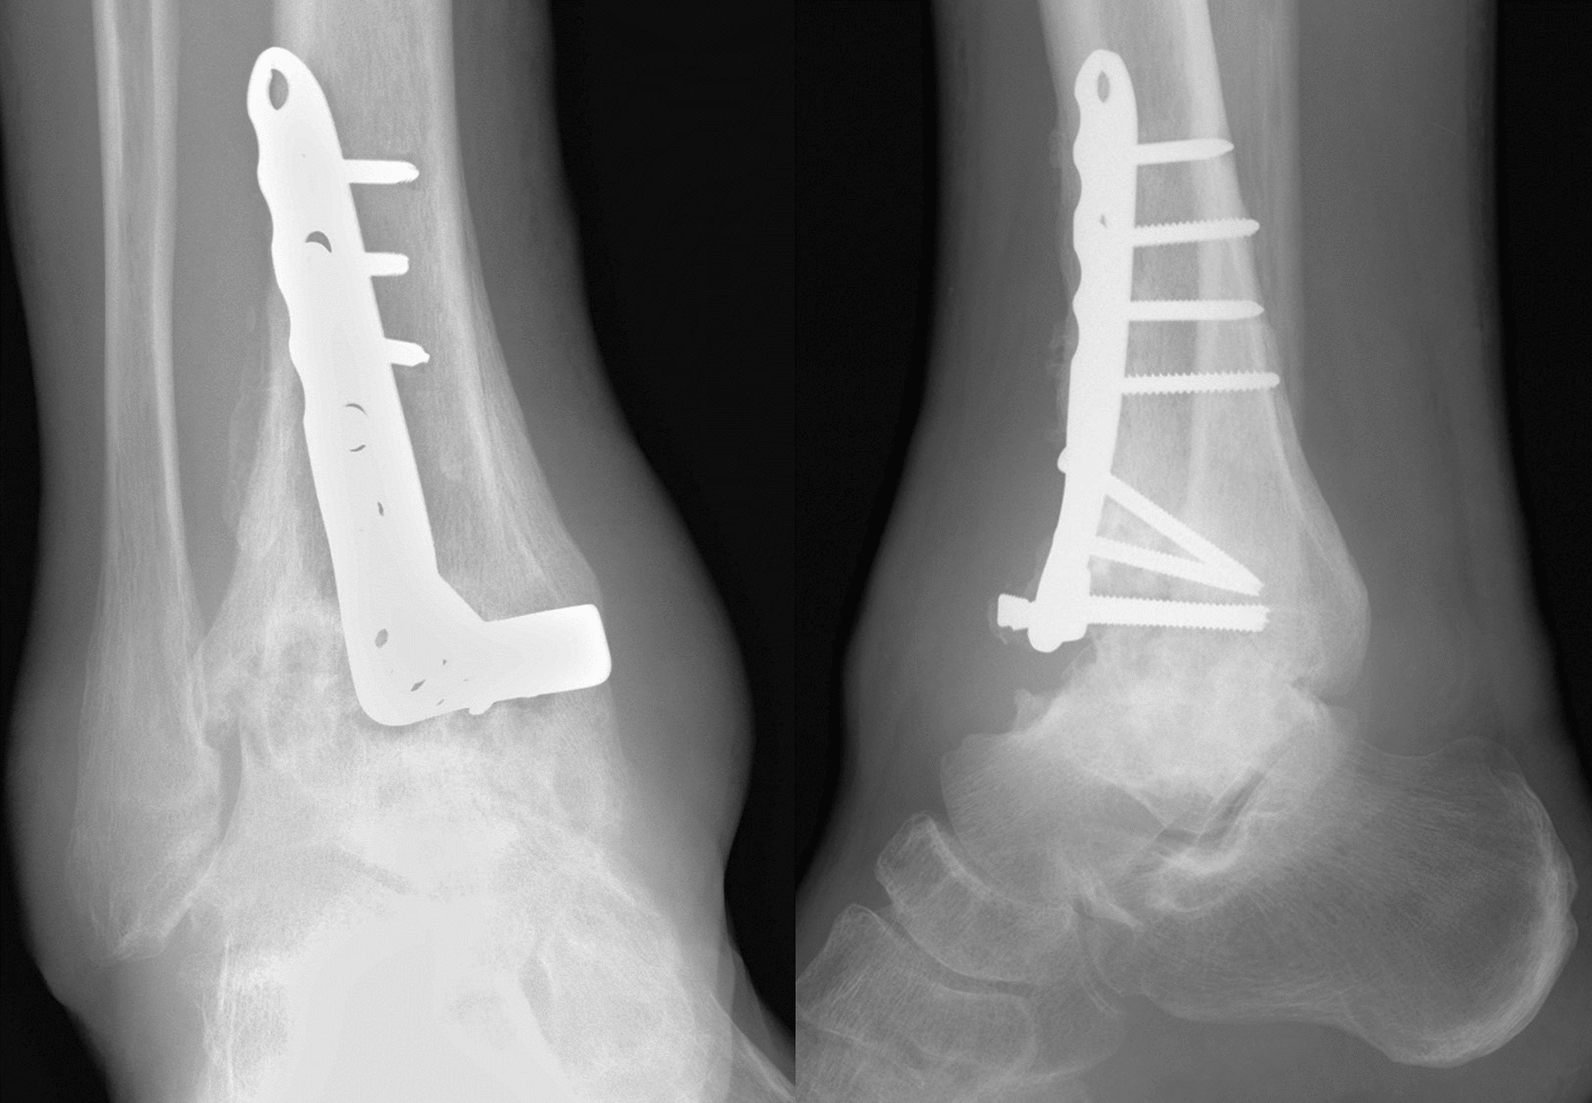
Plain radiographs of right ankle 10 months after osteosynthesis, demonstrating the rapid joint destruction subsequent to the procedure: (right) anteroposterior view and (left) lateral view
